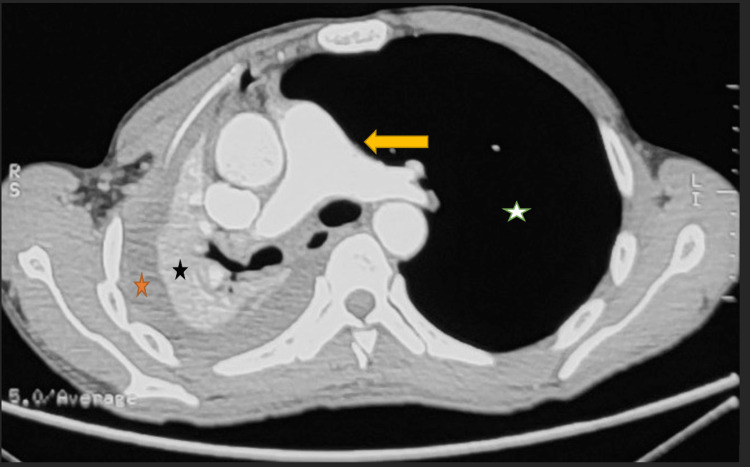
CT chest without contrast (transverse section) at the level of the lower thorax The image shows a collapsed lung (black star), a moderate right-sided hemothorax (orange star) along with a mediastinal shift to the same side (yellow arrow). There is a compensatory expansion of the left lung (white star) CT: computed tomography
